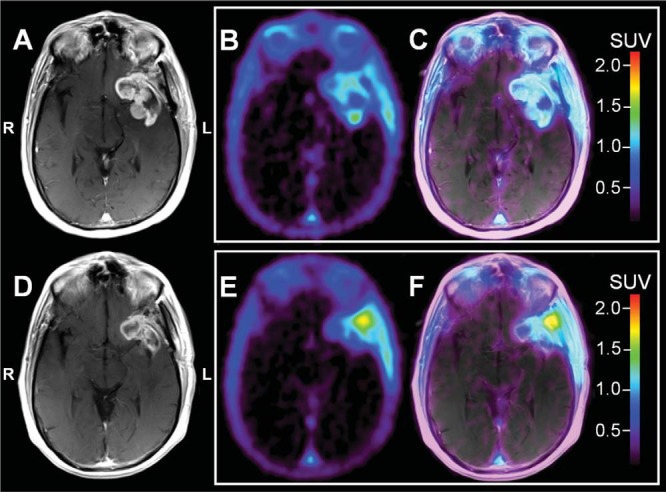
Patient ML-10 #3. Representative baseline (BL; A–C) and early-therapy assessment (ETA; D–F) contrast enhanced (CE) MRI, [^18^F]ML-10 standard uptake value (SUV), and PET/CE-MRI fusion images for a 60 year old male with a newly diagnosed left temporal GBM. All images are shown coregistered to the patient's BL CE-MRI. Before BL imaging, the patient underwent debulking. BL CE-MRI (A) shows a large residual component of peripherally enhancing, centrally cystic mass along the margins of the resection cavity within the right anterior temporal lobe, particularly involving the amygdala, and also extending anteriorly to the right orbitofrontal region. Compared with BL, the ETA CE-MRI (D) shows a reduction in CE. There is interval resolution of previously identified midline shift and sulcal effacement. On PET, the BL [^18^F]ML-10 SUV image (B) shows uptake in the CE region (C). The ETA [^18^F]ML-10 SUV image (E) shows decreased CE-associated uptake (F); however, there is increased [^18^F]ML-10 uptake in the region of the lateral border (E and F), possibly related to radiation therapy/chemotherapy associated changes.
